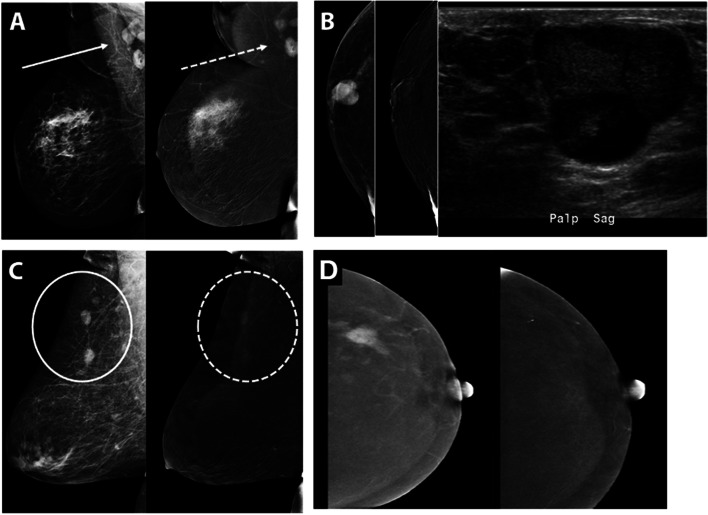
Associated Features of Malignancy and Other Entities. A Patient with multicentric breast cancer with enhancing metastatic disease to the right axilla (solid arrow corresponding to axillary lymph nodes on LE, dash arrow corresponding to enhancing axillary lymph nodes on RI). B 61-year-old man presented with palpable enhancing subareolar mass. Ultrasound correlate revealed a circumscribed hypoechoic mass with posterior enhancement, subsequently biopsied to reveal invasive ductal carcinoma with papillary features, for which a mastectomy was performed with curative intent. C 59-year-old woman with history of lymphoma presented with multiple oval enhancing masses in the breast (solid circle on LE, dash circle on RI) compatible with biopsy proven diffuse B cell lymphoma. D 56-year-old woman with known infiltrating ductal carcinoma, shown before and after neoadjuvant chemotherapy RI CEM pre-treatment image shows an enhancing spiculated mass with resolution of enhancement and mass on post-treatment RI (clip indicates an area of biopsy-proven malignancy)
